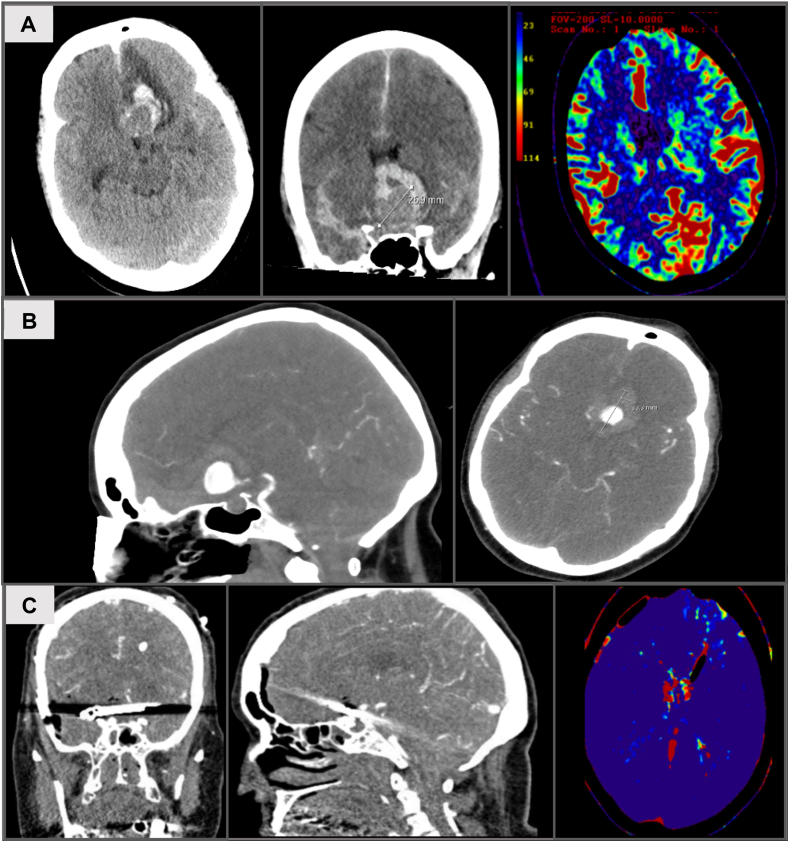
Case One.* Preoperative (A–B) and postoperative (C) CT, CTA and perfusion.
